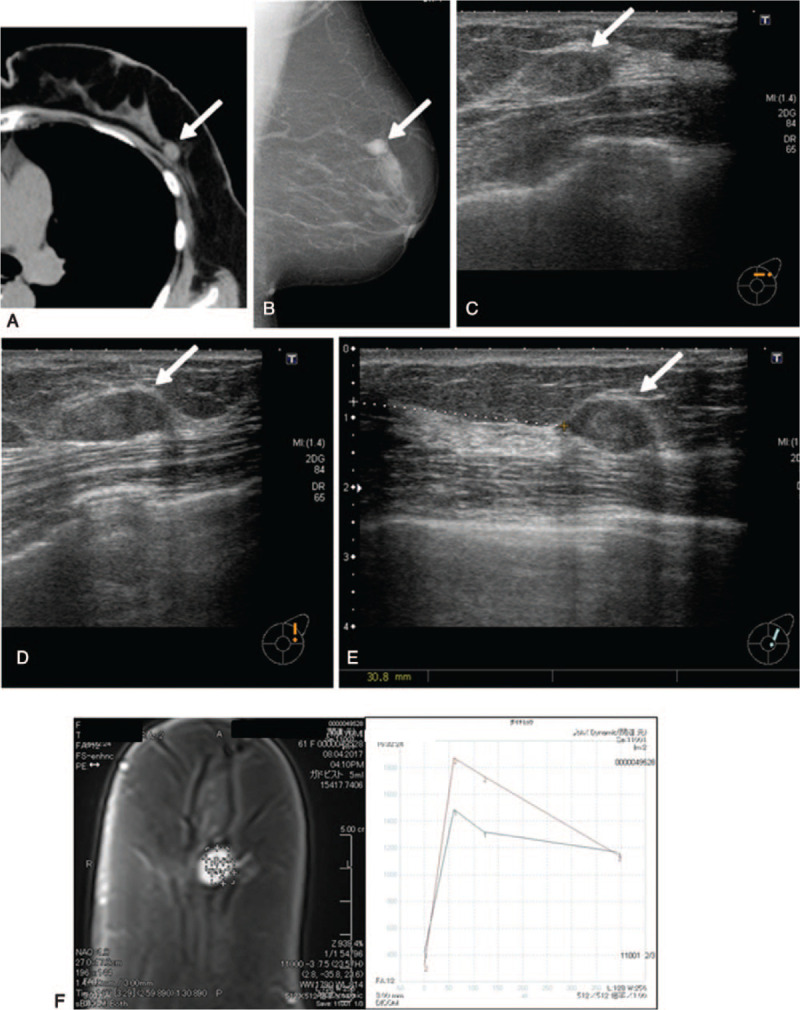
Imaging findings. (a) Chest CT shows a 12-mm ovoid homogeneous mass with defined margin in the left breast C area (arrow). (b) Mammography shows a 16-mm high-density tumor without calcification (arrow). (c–e) US reveals a thick, disk-shaped, slightly inhomogeneous low-echogenic tumor (arrow) with a defined margin with lateral echo, gained echo, and no halo sign. The tumor measures 15.8 mm × 14.1 mm × 7.5 mm and is slightly larger especially in thickness than that measured 5 years ago. The nipple-to-tumor distance was 31 mm in the 1 o’clock direction. (f) The time–intensity curve of dynamic MRI shows a malignant pattern with rapid enhancement and washout.
